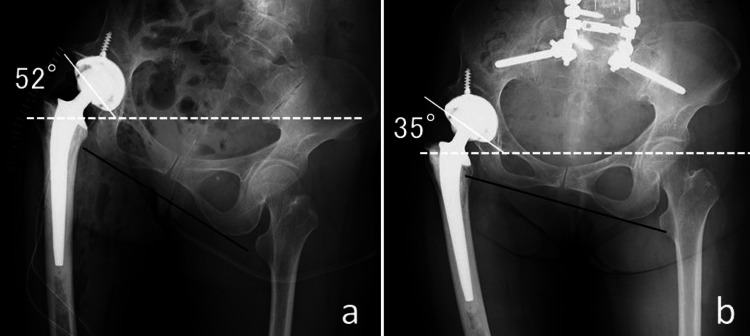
Radiographs of the hip joints before and after spinal correction surgery. After spinal correction surgery, the inclination angle for the horizontal line has changed from 52° to 35°, whereas the angle for the line connecting both teardrops is stable.
